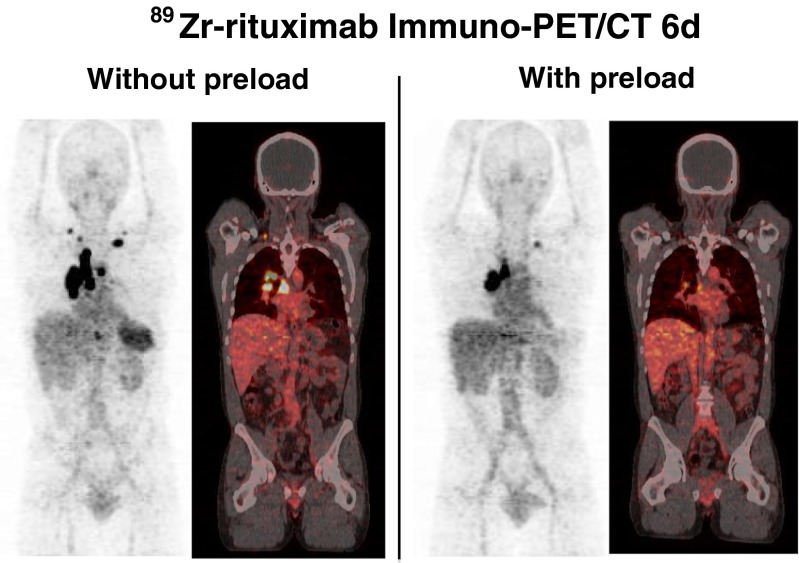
^89^Zr-rituximab immuno-PET/CT images in patient 4 (with CD20+ B-cell depletion) obtained 6 days after injection without and with a preload of unlabelled rituximab show consistently better tumour targeting without the preload
